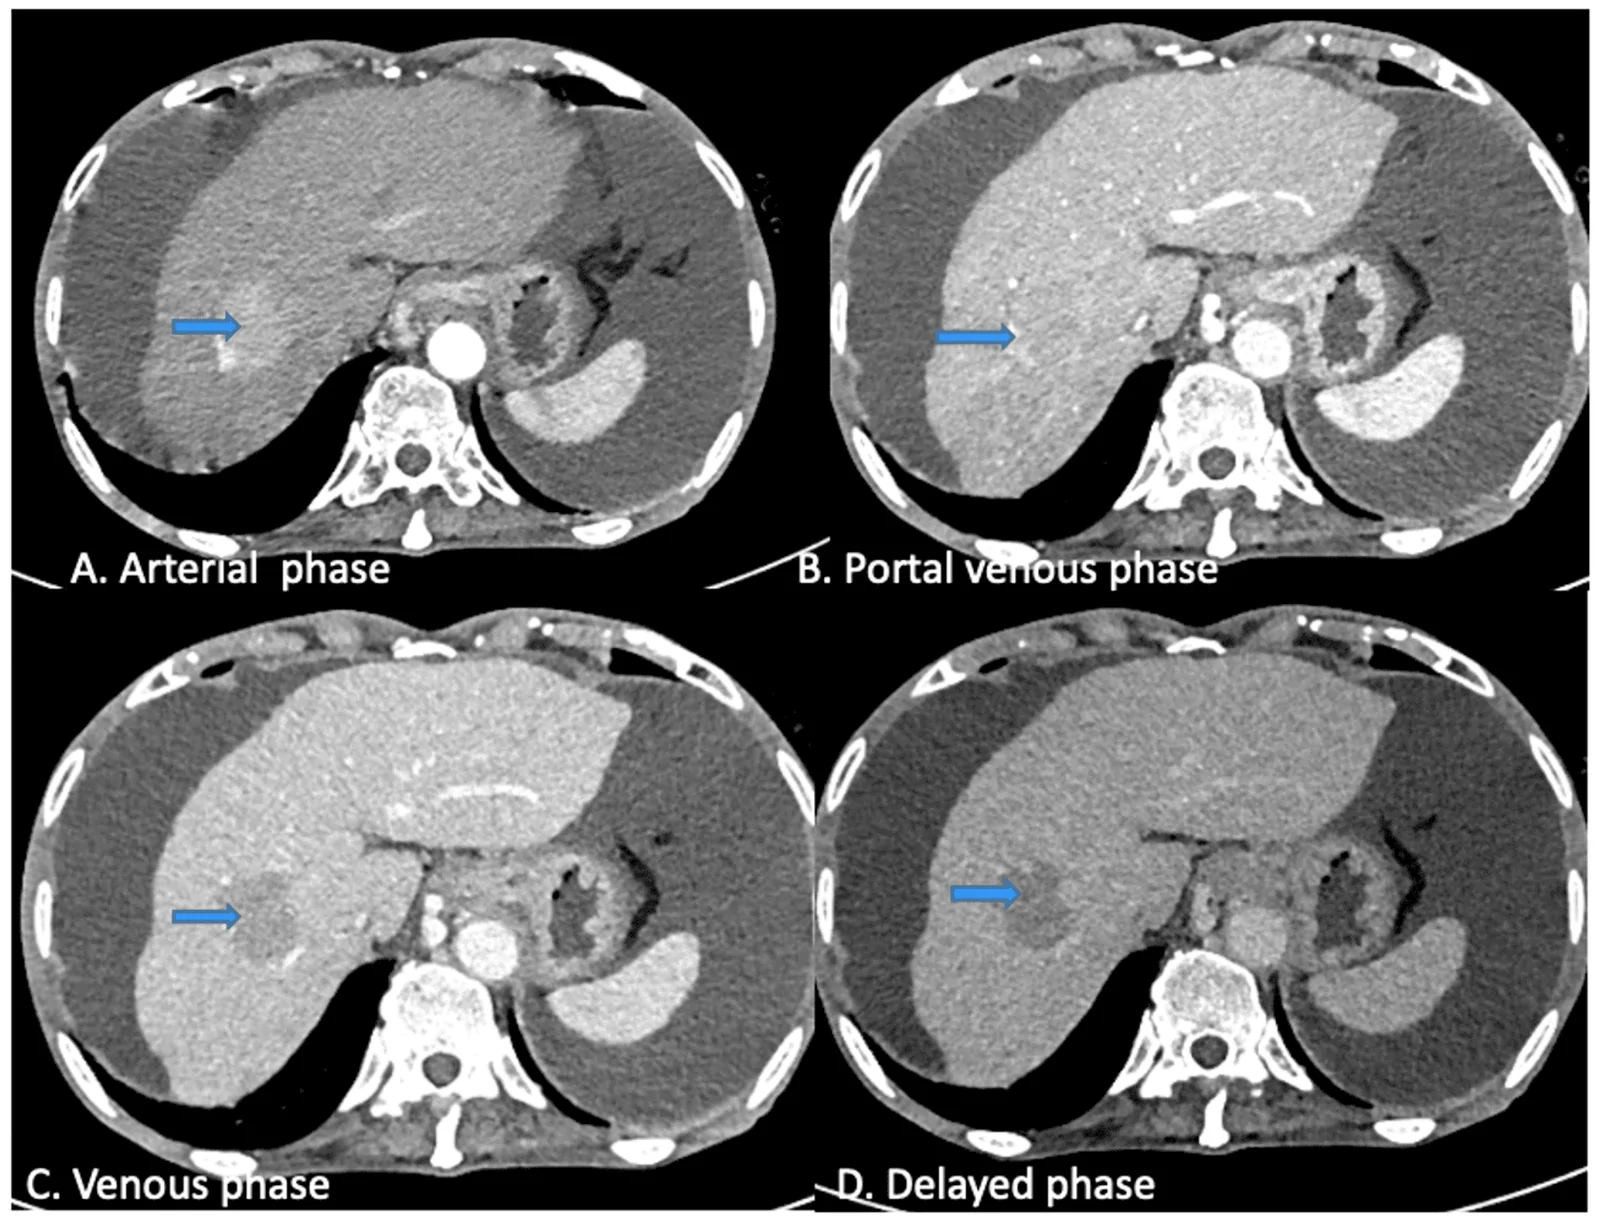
LR-5 observation on multiphasic contrast-enhanced CT. Axial multiphasic contrast-enhanced CT images obtained during the arterial (A), portal venous (B), venous (C), and delayed (D) phases demonstrate a well-defined hepatic observation measuring >2 cm (blue arrow). The observation demonstrates nonrim arterial phase hyperenhancement, nonperipheral washout on the portal venous, venous, and delayed phases, and an enhancing capsule on the delayed phase. Background findings include chronic liver disease with ascites. These major imaging features are consistent with an LR-5 observation (definitely hepatocellular carcinoma according to the Liver Imaging Reporting and Data System (LI-RADS)).
